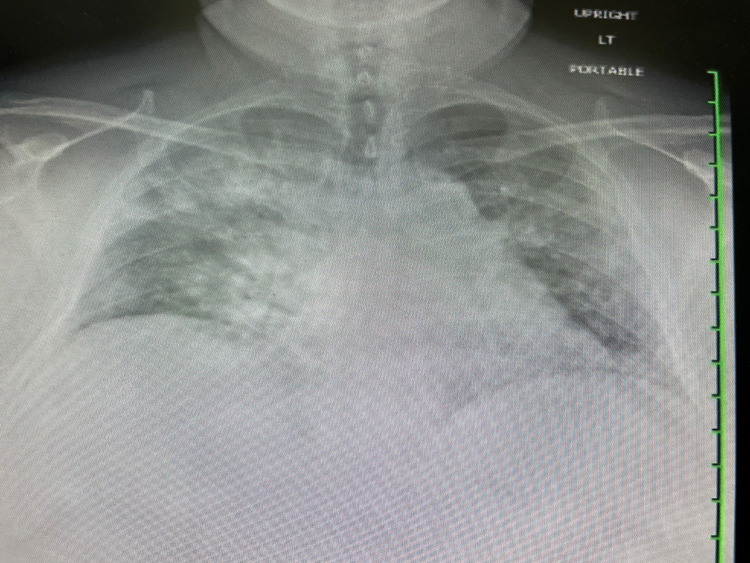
Diffuse bilateral airspace opacities noted on the chest X-ray
